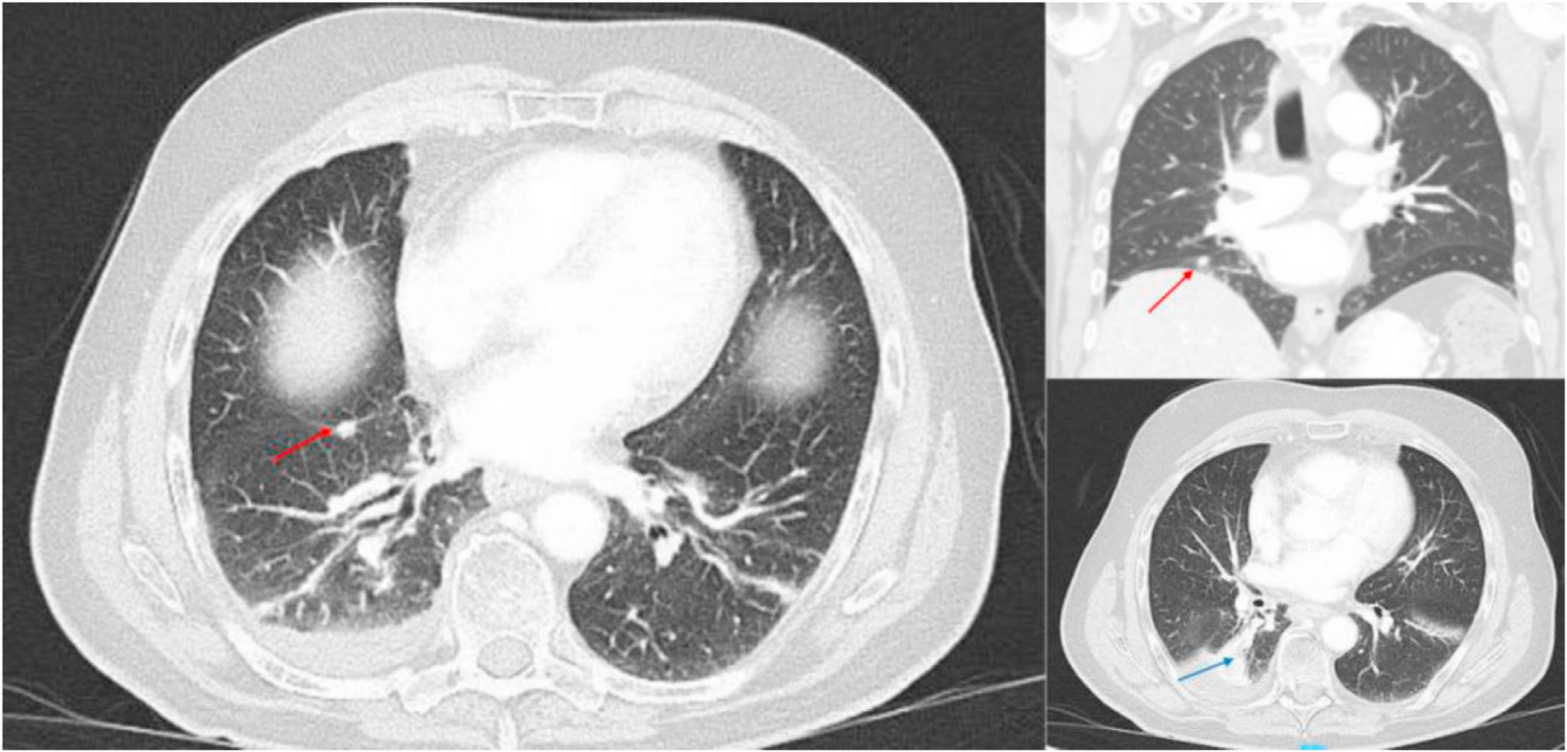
Case 2. Thorax and abdominal CT scan (August 31, 2021). Signs of radiation pneumonitis primarily affecting the upper segment of the right inferior lobe (blue arrow). Nodule of 6.8 mm in the same lobe (red arrow). No other pathological findings.PET-CT showed right pleural and retroperitoneal progression, as well as progression in the right pleura, diaphragm, and right supraclavicular lymph nodes (Fig. 3). None of those findings were previously identified by the CT scan performed.
